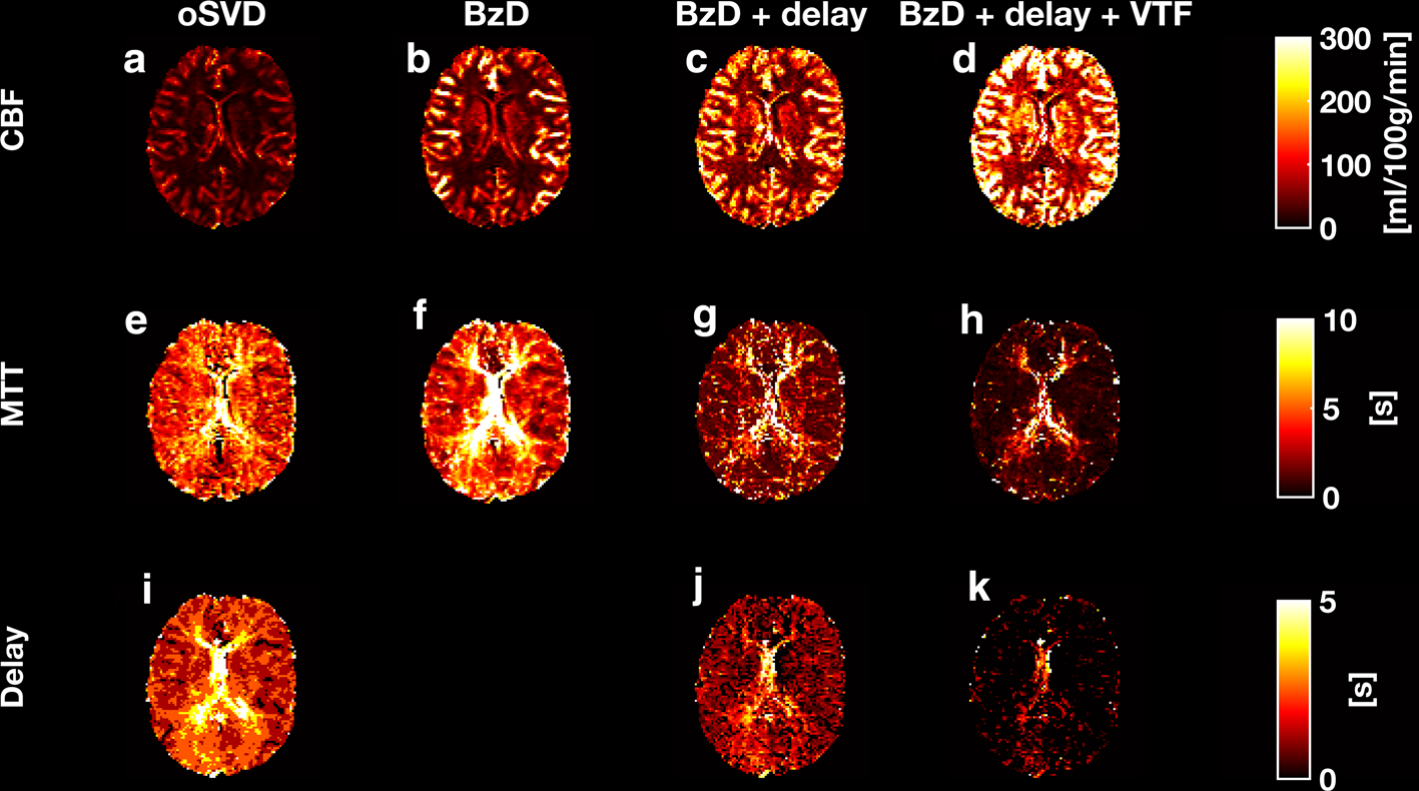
CBF, MTT and delay maps from the analysis of in vivo DSC-MRI data from a healthy volunteer using oSVD, BzD, BzD with delay correction and BzD with correction for both delay and dispersion
